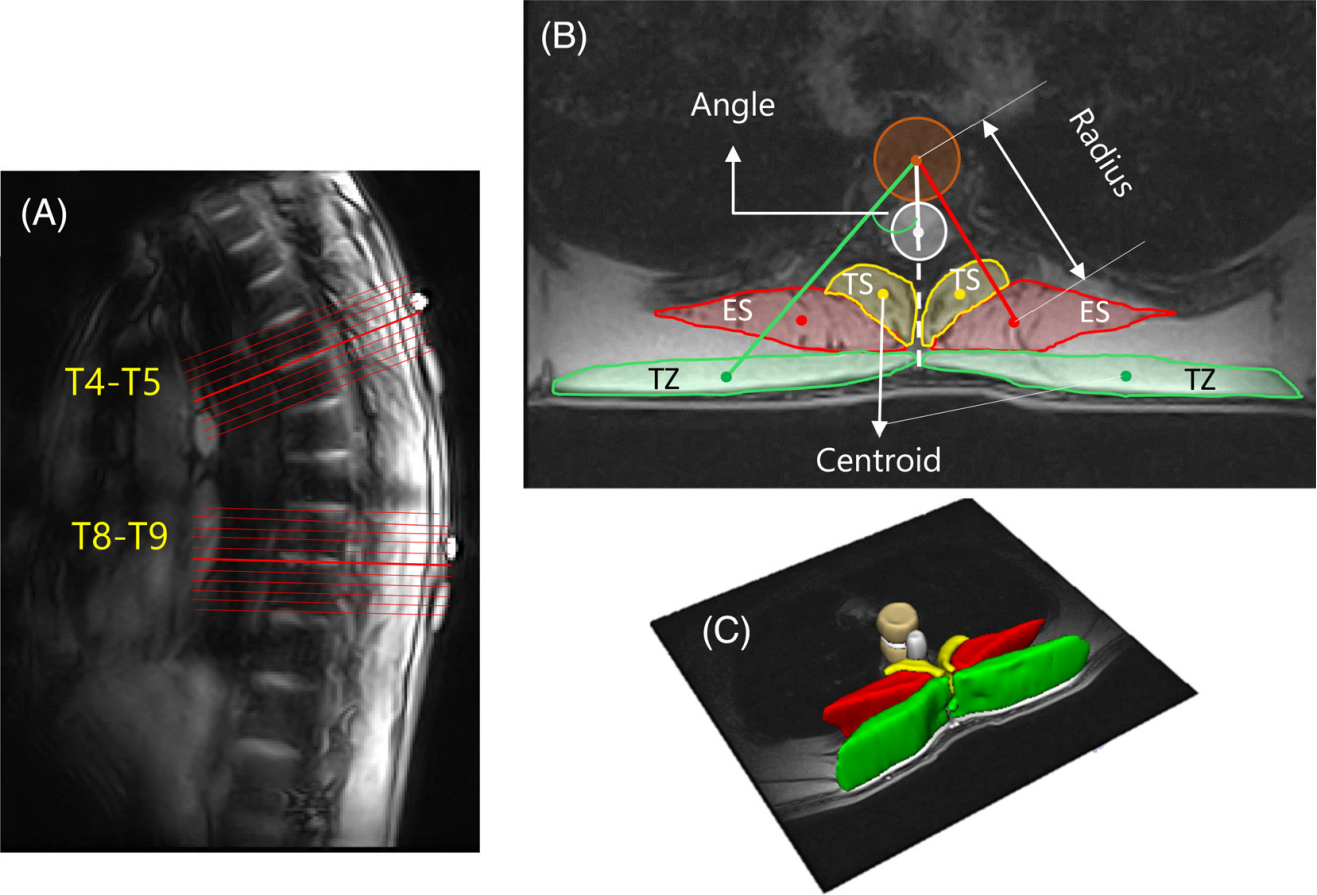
A, Sagittal MR image showing the orientation of the parallel slice stack for thoracic levels T4 to T5 (9 slices) and T8 to T9 (11 slices). B, Image analysis measurements of 2D muscle CSA and position (radius and angle). The brown colored circle represents the vertebral body, the white colored circle represents the vertebral canal. C, 3D geometry obtained from series of 2D muscle segmentations. ES, erector spinae; TS, transversospinalis, TZ, trapezius
